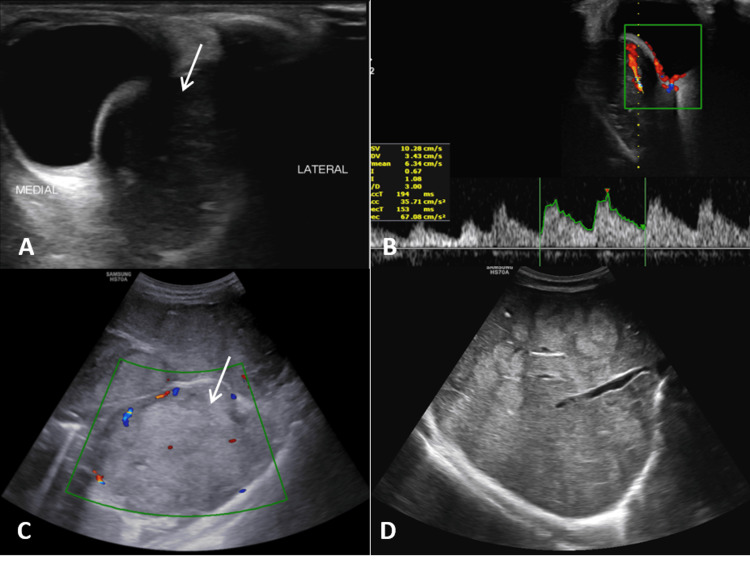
Ultrasound scan images of the left orbit (A, B) reveal a heterogeneously hypoechoic retro-bulbar mass lesion (arrow) showing vascularity on color Doppler with a low resistance waveform on spectral Doppler. Ultrasound of the abdomen and pelvis (C, D) shows a large heterogeneously hyperechoic lesion in the left renal fossa, abutting the spleen supero-laterally and the left lobe of the liver medially (C, arrow). Additionally, multiple round to oval hyperechoic focal lesions diffusely involving the liver parenchyma, most with peripheral halos, are observed (D). These findings are consistent with retro-bulbar, hepatic, and left renal fossa metastases.
